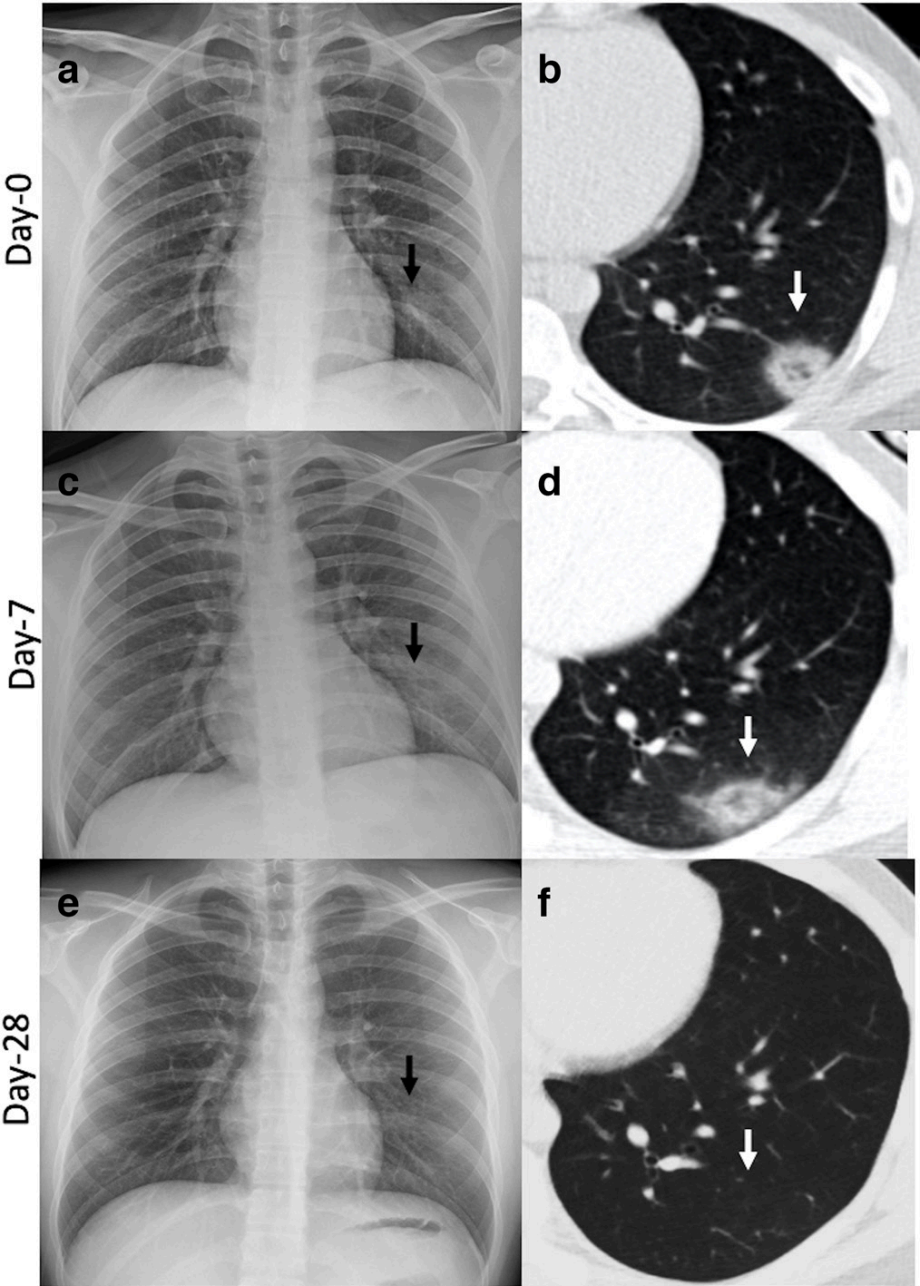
Patient with COVID-19 pneumonia getting serial imaging. Chest X-ray (A) and chest CT (B) obtained 2 weeks after diagnosis showing faint left paracardiac opacity (black arrow in A) which was confirmed as a central GGO with peripheral consolidation (reverse-halo sign, white arrow B, D). Follow-up imaging a week later showing slightly decreased density on CXR (C, black arrow) with minimally decreased peripheral consolidation on CT (white arrow, D). CXR (E) and CT (F) obtained 28 days after initial imaging showing complete resolution of the disease. CXR, chest radiographs; GGO, ground-glass opacity.
